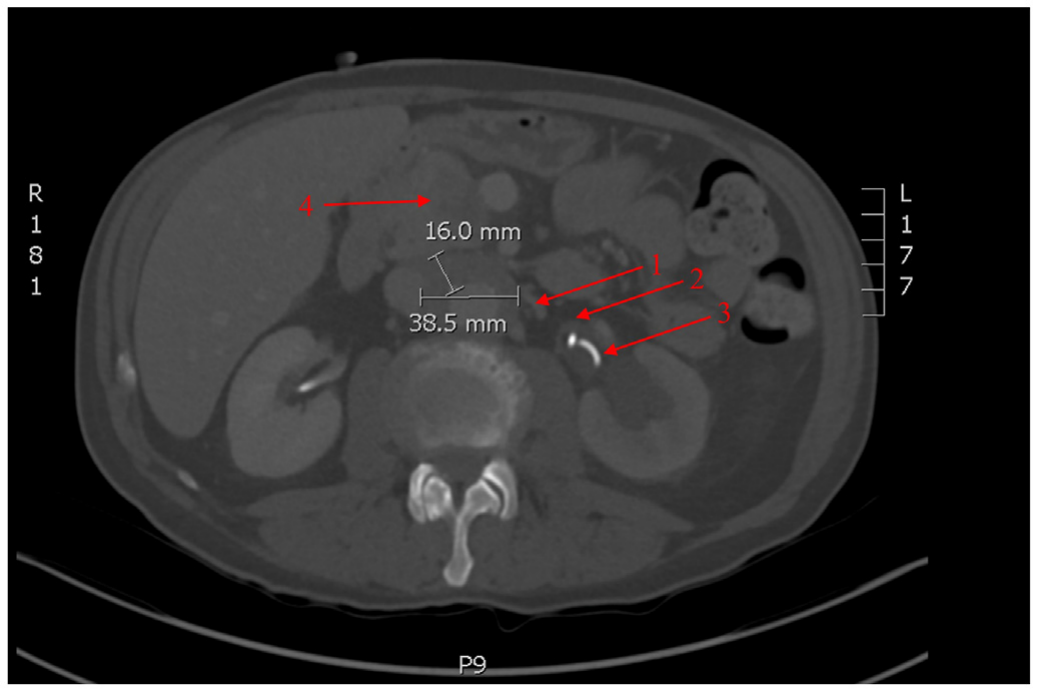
Computed tomography (CT) angiography of the abdomen from September 2022. Six months after the discontinuation of nivolumab. Infrarenal aortitis (1) with stable maximal aortic wall thickness measured at 16 mm and axial diameter 38.5 mm with persistent retroperitoneal fibrosis (2). Left ureteric stent seen (3). Additional findings of a necrotic pancreatic head mass (4) and hypodense liver lesions (not visible in this cross-section).
